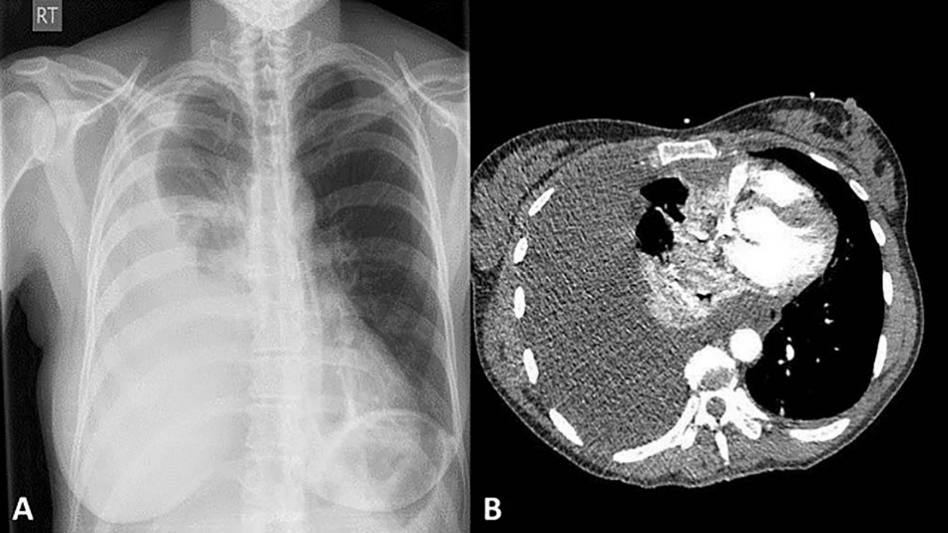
A postero-anterior plain CXR showing a large right-sided pleural effusion (A); a contrast-enhanced CT scan showing large right-sided pleural effusion causing right lung collapse and left mediastinal shift with compression of the right atrium; note the intact ribs and vertebra (B).
